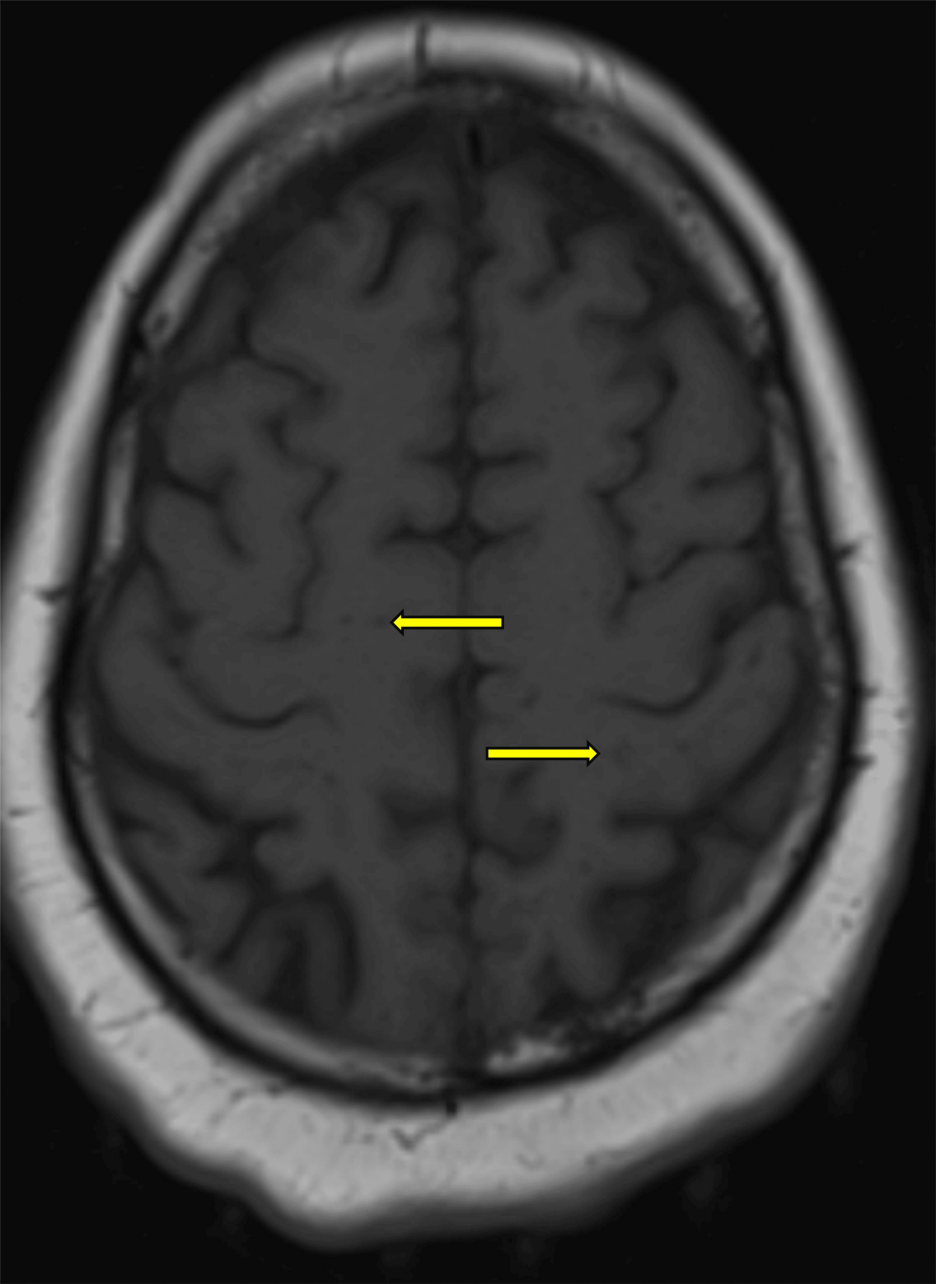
Multiple nodules (arrows) noted throughout the brain, consistent with tuberculosis granulomas in MRI.
